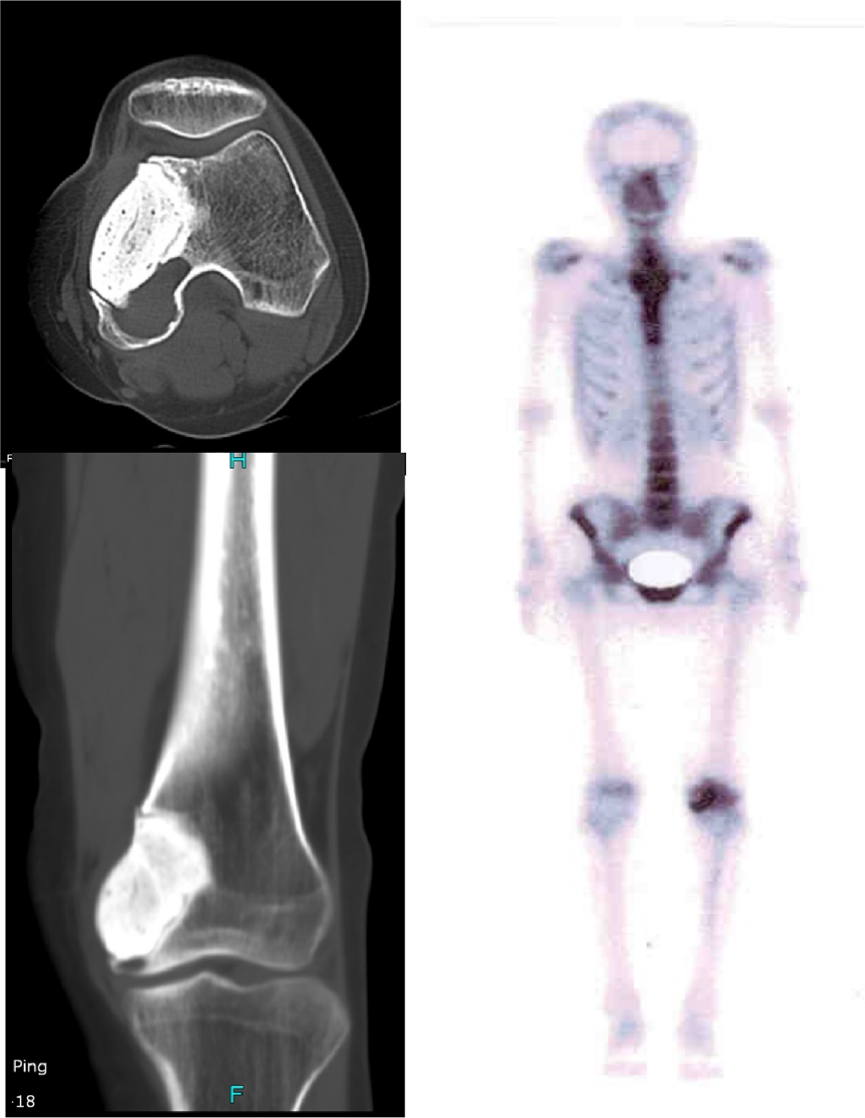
18 months after the first surgery, CT and bone scan found local recurrence was found at follow-up visit.
